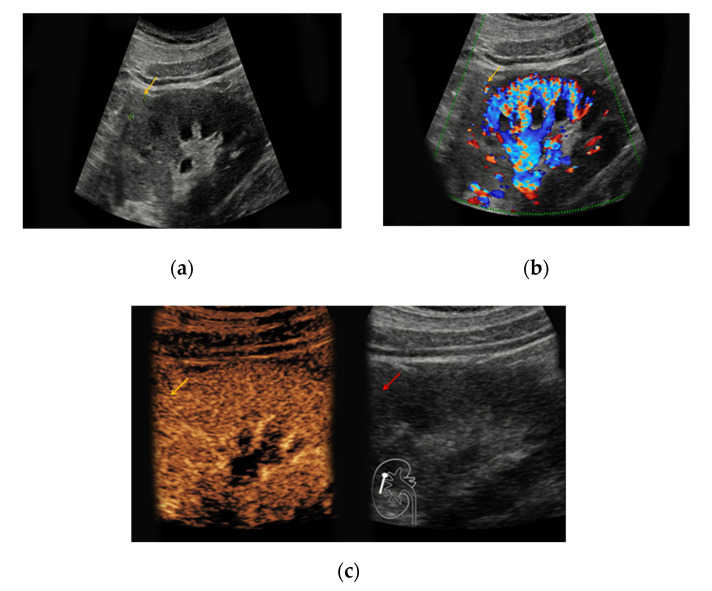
Renal oncocytoma in the right kidney with a diameter of 1.2 cm. (a) In native B-mode, the lesion presents as slightly hyperechoic (yellow arrow); (b) the lesion shows discrete vascularization in Doppler mode. (c) Upon intravenous application of SonoVue^®^, the lesion shows no signs of hyperenhancement.
